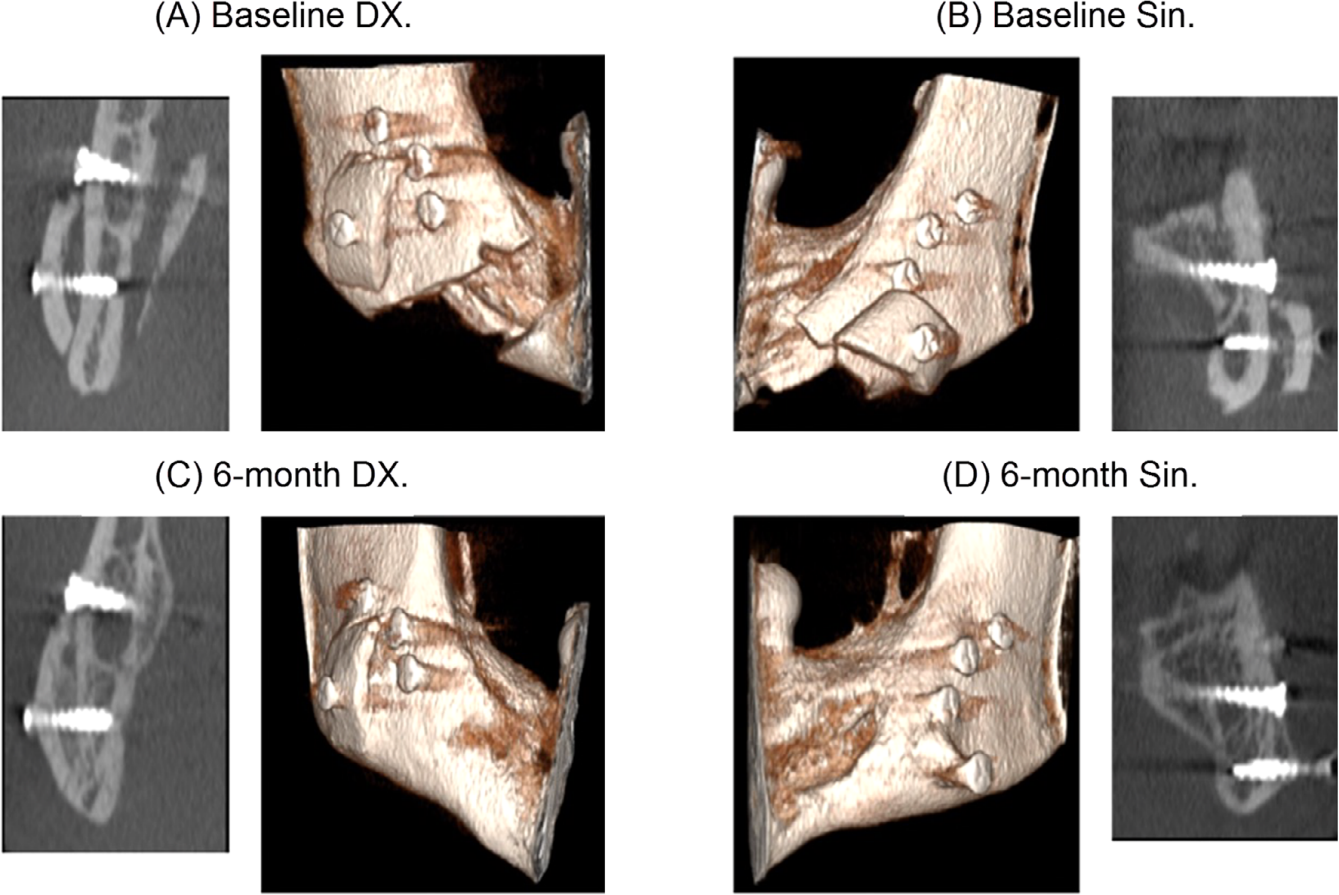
Three‐dimensional volumes and coronal cone beam computed tomography X‐ray slices of patient ID 5421. At the top images from the baseline and below from the 6‐month control. Right images show the left side of the mandible (Sin) and left images show the right side of the mandible (Dx).
